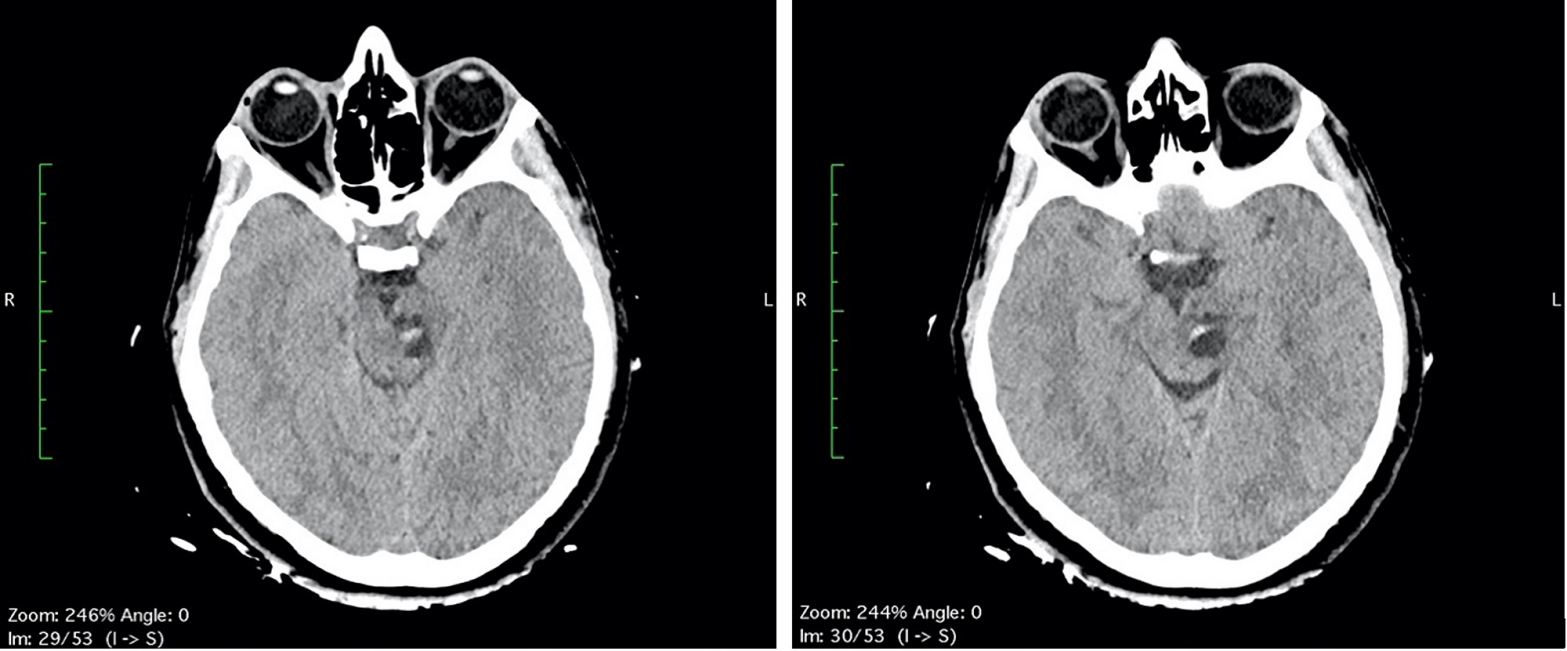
(A-B) Head CT scan: a multiloculated cyst in the left midbrain with eccentric hyperdensity, hematic-like.
